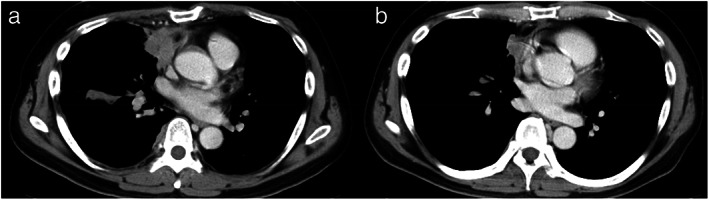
Radiographic results before and after initial lenvatinib. (a) Computed tomography scan showing a primary lesion in the anterior mediastinum before initial lenvatinib. (b) Tumor shrinkage 2 months after initial lenvatinib
